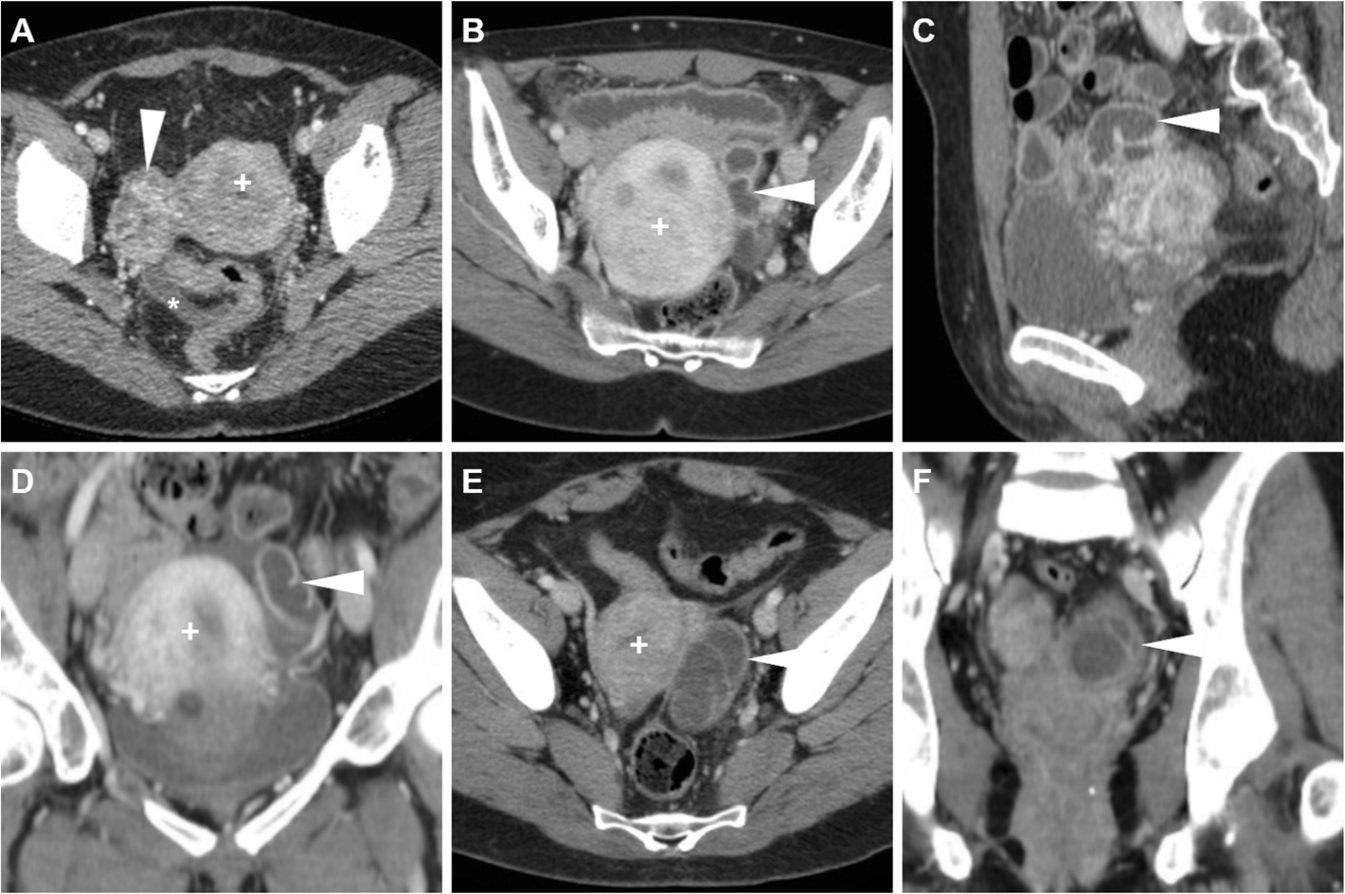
CT appearances in three different patients with a clinical diagnosis of PID. a Heterogeneously enhancing, unilaterally enlarged right ovary (arrowhead). Note uterus (plus sign), mild cul-de-sac effusion (asterisk). b–d Multiplanar images of tubular-shaped left salpingitis (arrowheads), distended with prominent mural enhancement. e–f Enlarged, septated left ovary (arrowheads) with thickened walls consistent with early tubo-ovarian abscess (TOA)
